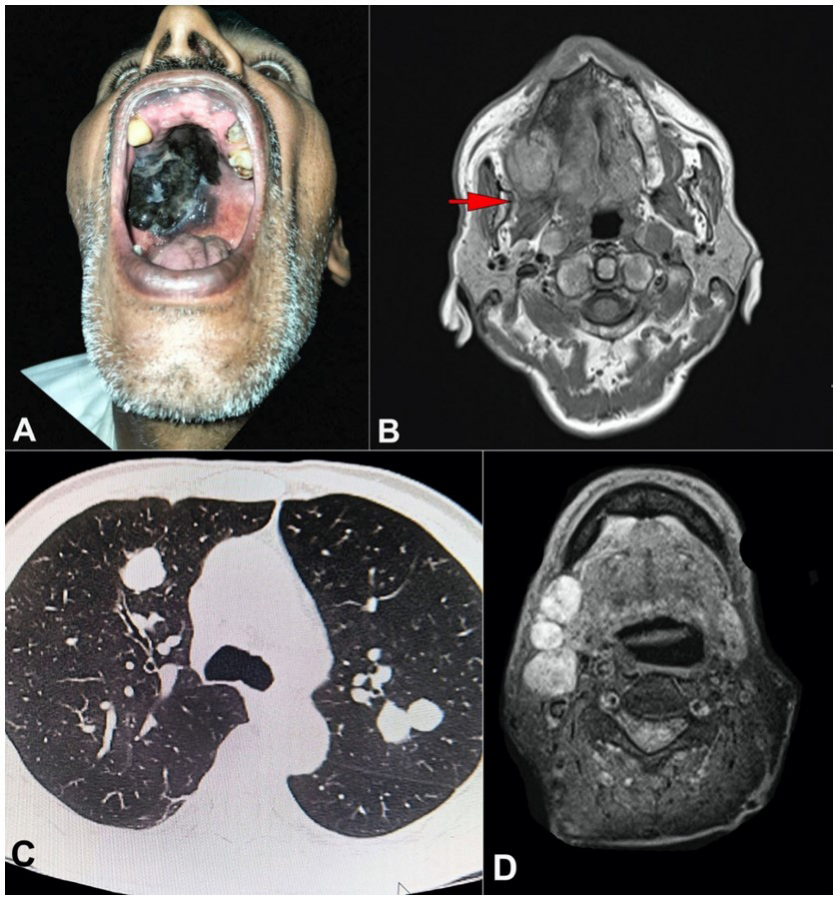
A – Gross view of the oral examination shows a blackish mass in the hard palate; B – Contrast-enhanced magnetic resonance imaging (CE-MRI) shows a well-defined irregularly shaped T2 hypointense lesion (3.7x3.2x2.0 cm) at the right side of hard palate; C – Axial thoracic HRCT, lung window image, showing - well defined round soft tissue attenuation lesions are seen in the anterior segment of the right upper lobe, and the apical-posterior segment of the left upper lobe; D – T1 fat-saturated images show enlarged cervical lymph nodes in the right level Ib and II station.
